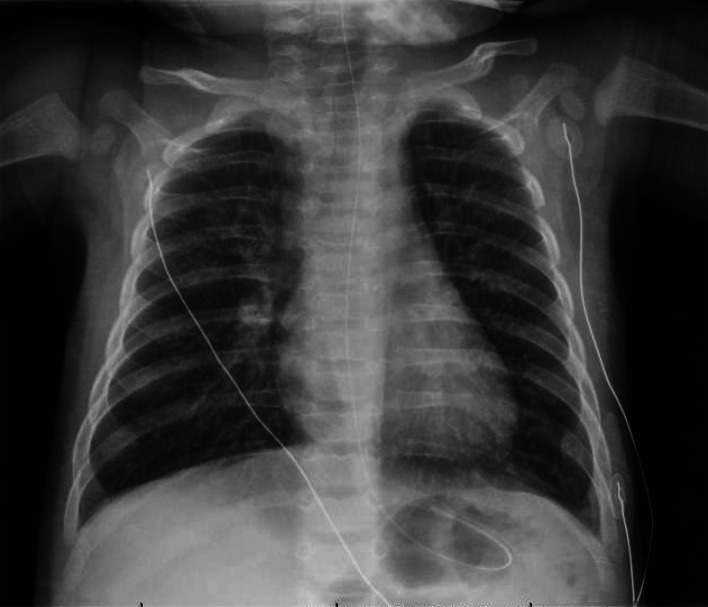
Posteroanterior chest radiograph, March 22, 2020 (Illness Day 6, Hospital Day 2); increased interstitial markings with some linear and granular opacities noted bilaterally without confluent airspace opacities.
